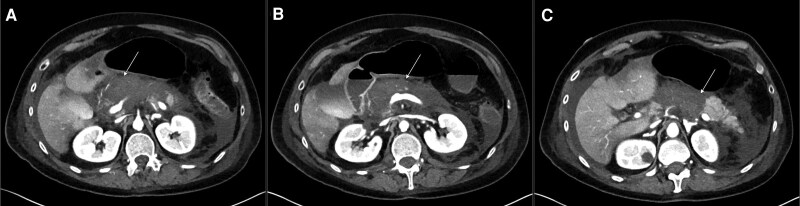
(A-C) Computed tomography scan of the abdomen and pelvis demonstrating absence of parenchymal enhancement in the pancreatic body, pancreatic neck, and a portion of the pancreatic head, suggestive of worsening necrotizing pancreatitis. Increased peripancreatic inflammatory changes and ill-defined peripancreatic fluid. Arrows pointing to the pancreas.
